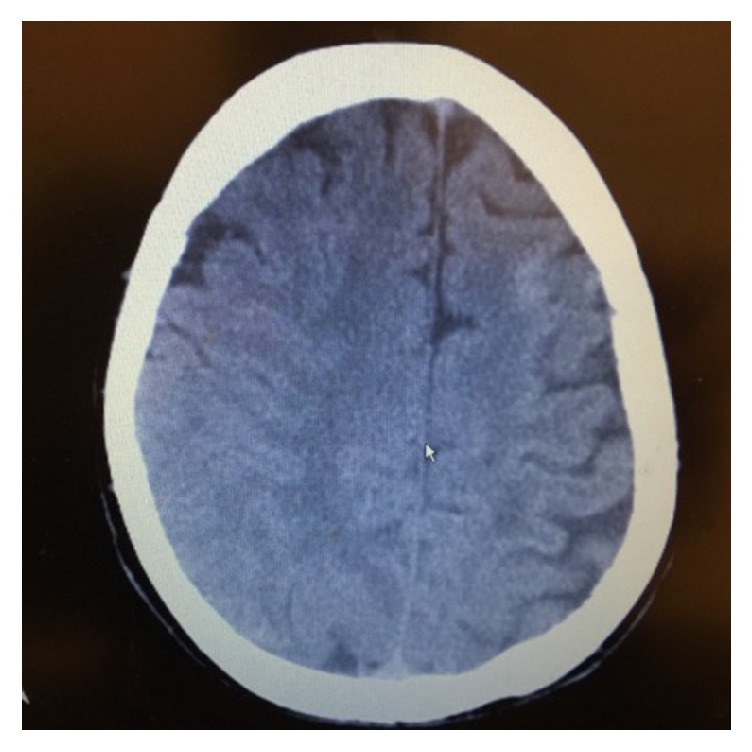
CT head showing left frontal hyperdense lesion on left frontal region.
